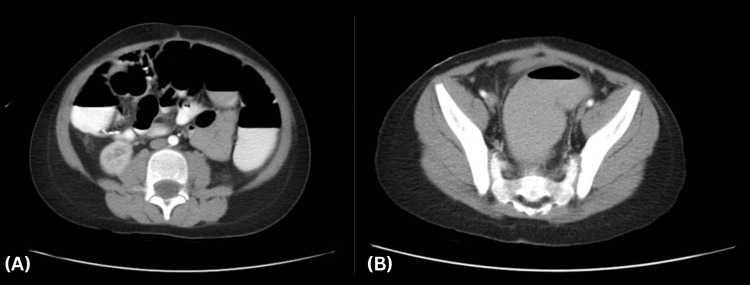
Third day of admission. Axial abdominal and pelvis CT scan demonstrates diffuse colonic dilation extending to the rectum without a transition point or evidence of mechanical obstruction, consistent with acute colonic pseudo-obstruction (ACPO). (A) Air-fluid levels are seen within the colon containing oral contrast. The cecum measures 5.0 cm, with surgical clips in place from the prior appendectomy. (B) Distended rectosigmoid colon with no transition point.
